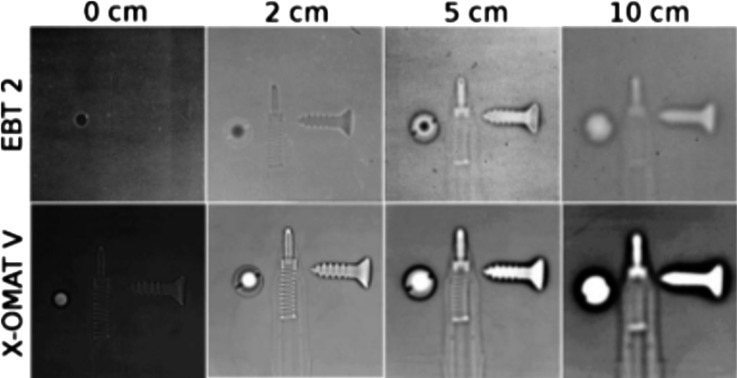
Radiographs of a pen tip and screw acquired with radiochromic film and varying air gap offsets, published in 2011.^34^ Images with two types of radiochromic film are presented: with EBT 2 (Ashland Inc., Covington, KY) and with X-OMATV (Carestream Health, Rochester, NY). Reprinted from Seco and Depauw^34^ with permission from the American Association of Physicists in Medicine.
